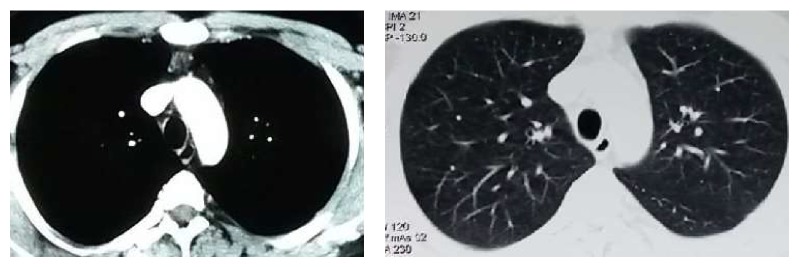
CT sections at the 6th month: tracheal lumen is normal.
